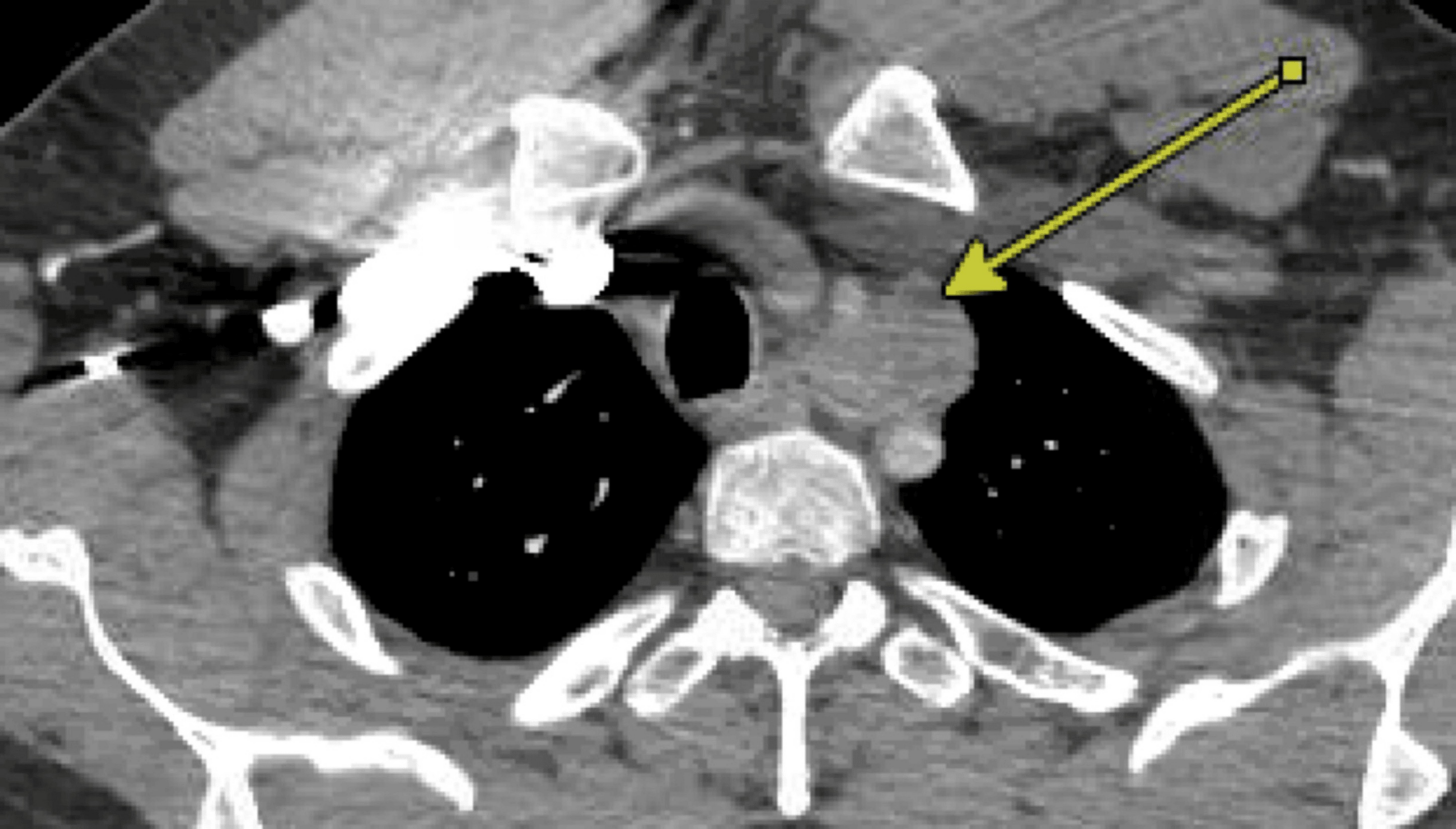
Case 2: CT scan of the chest Arrow pointing at the lesion near the aorta
